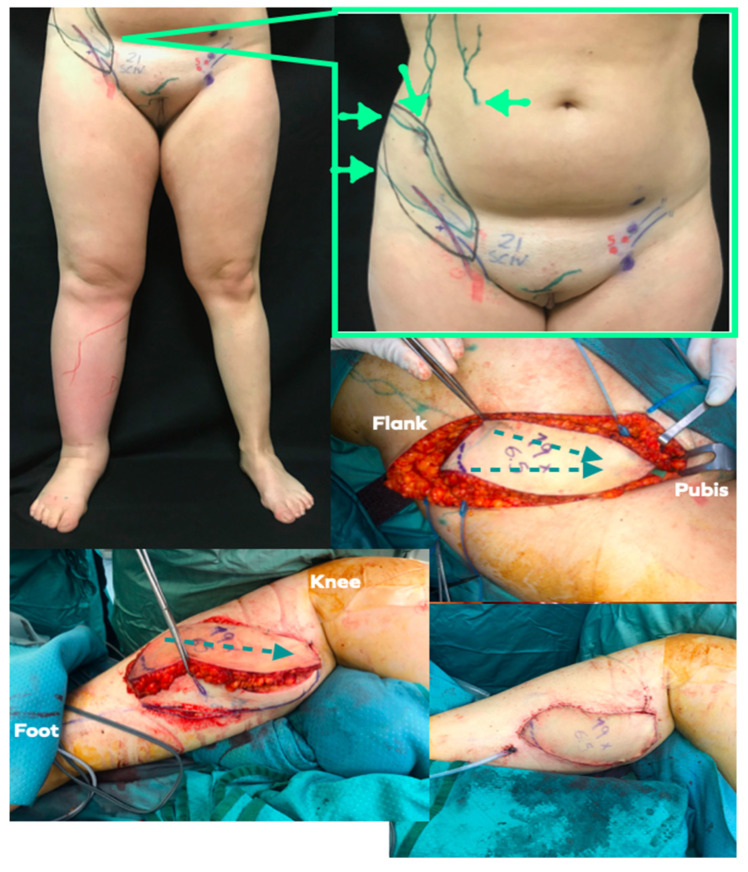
LYST case example. Above, left: Preoperative picture of 30-year old female patient suffering from primary lower limb lymphedema. Lymphoscintigraphy showed dermal backflow in the no tracer progression. Above, right: Preoperative planning markings were conducted with US and ICG-lymphography. The flap (flap margin is marked in black) has been oriented along the main axis of lymphatic channels (green lines) and SCIA (blue and red lines). Green arrows show the ICG injection points to highlight the lymphatic channels draining toward working inguinal nodes (green dots). Middle, right: Flap elevated according to preoperative planning. The green dotted line shows the lymph axiality as already highlighted preoperatively. Below, left: The flap has been anastomosed to the medial sural vessel and inset respecting lymph axiality. Lymphedematous skin and soft tissue have been resected to introduce more healthy soft tissue (i.e., flap) and resect the lymphedematous tissue. Below, right: Final picture.
